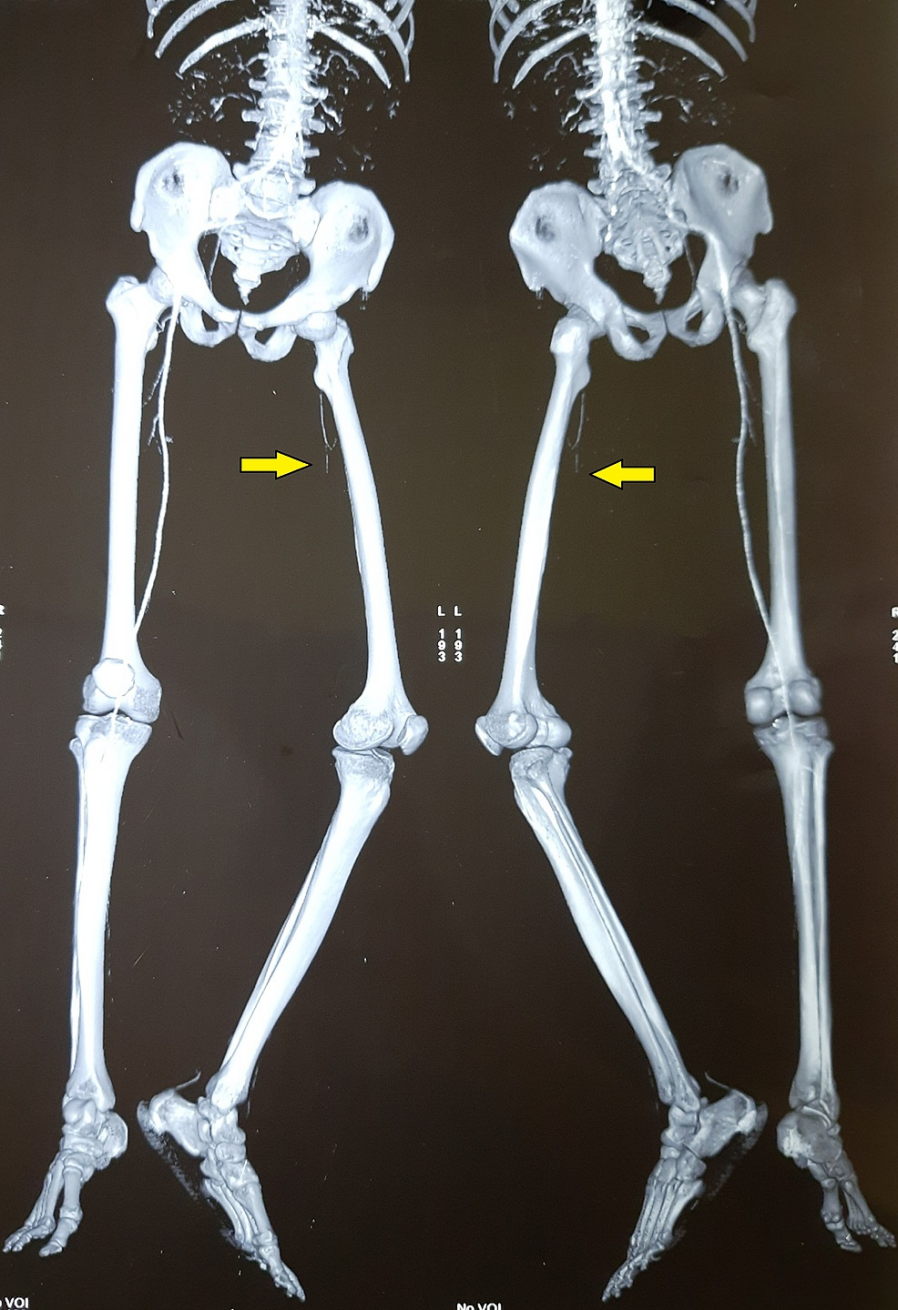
It is a surface shaded image of CTA depicting complete blockage blood supply to the left lower limb from the level of common iliac artery (Arrow) CTA= CT Angiography
